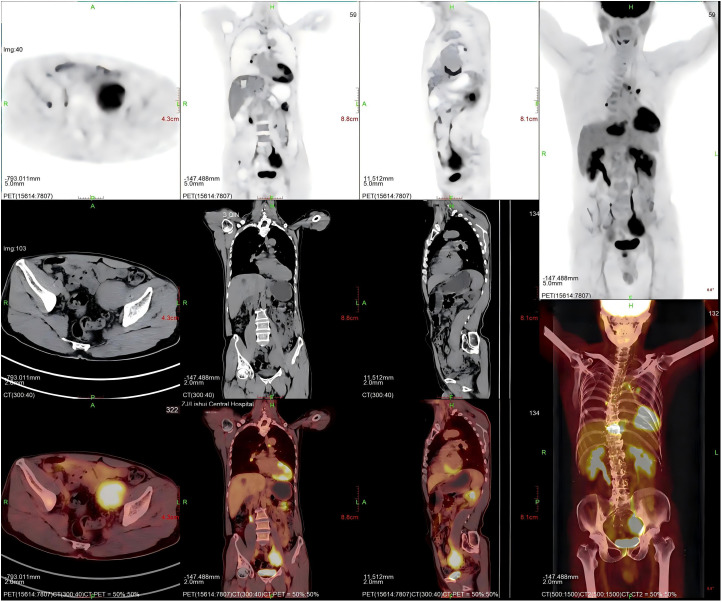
PET-CT revealed hypermetabolic masses in the anterior mediastinum, adjacent to right cardiophrenic angle, in the retroperitoneum, and adjacent to the left iliac vessels in the pelvis.
